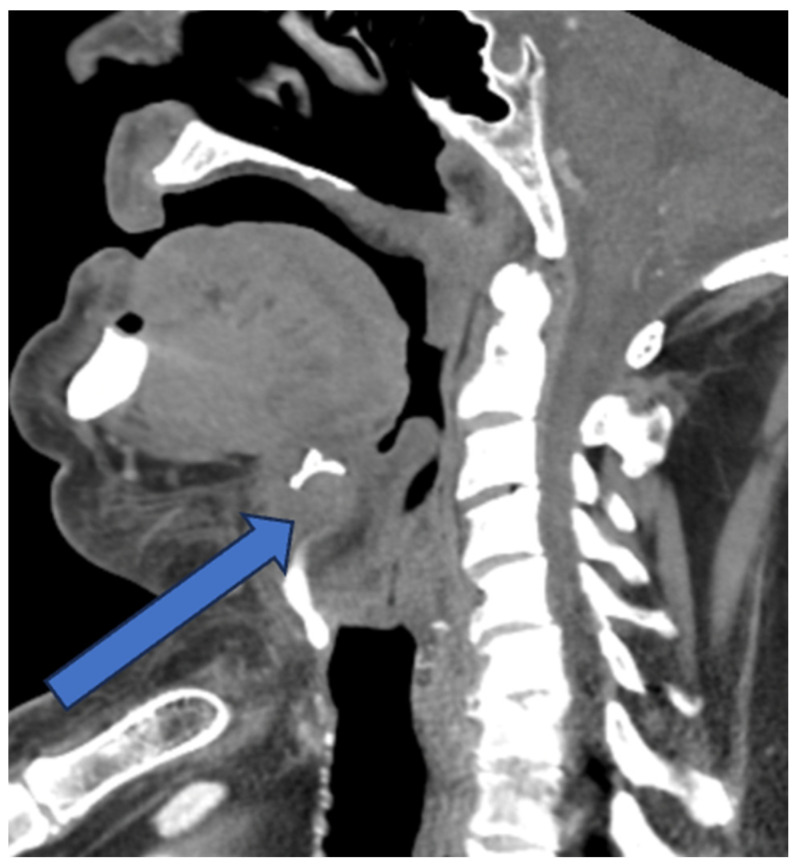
Sagittal view on a CT scan showing unspecific inflammatory lesion of the soft tissue. Arrow pointing to the thyrohyoid membrane and the hyoid bone with peripheral tissue inflammation.
